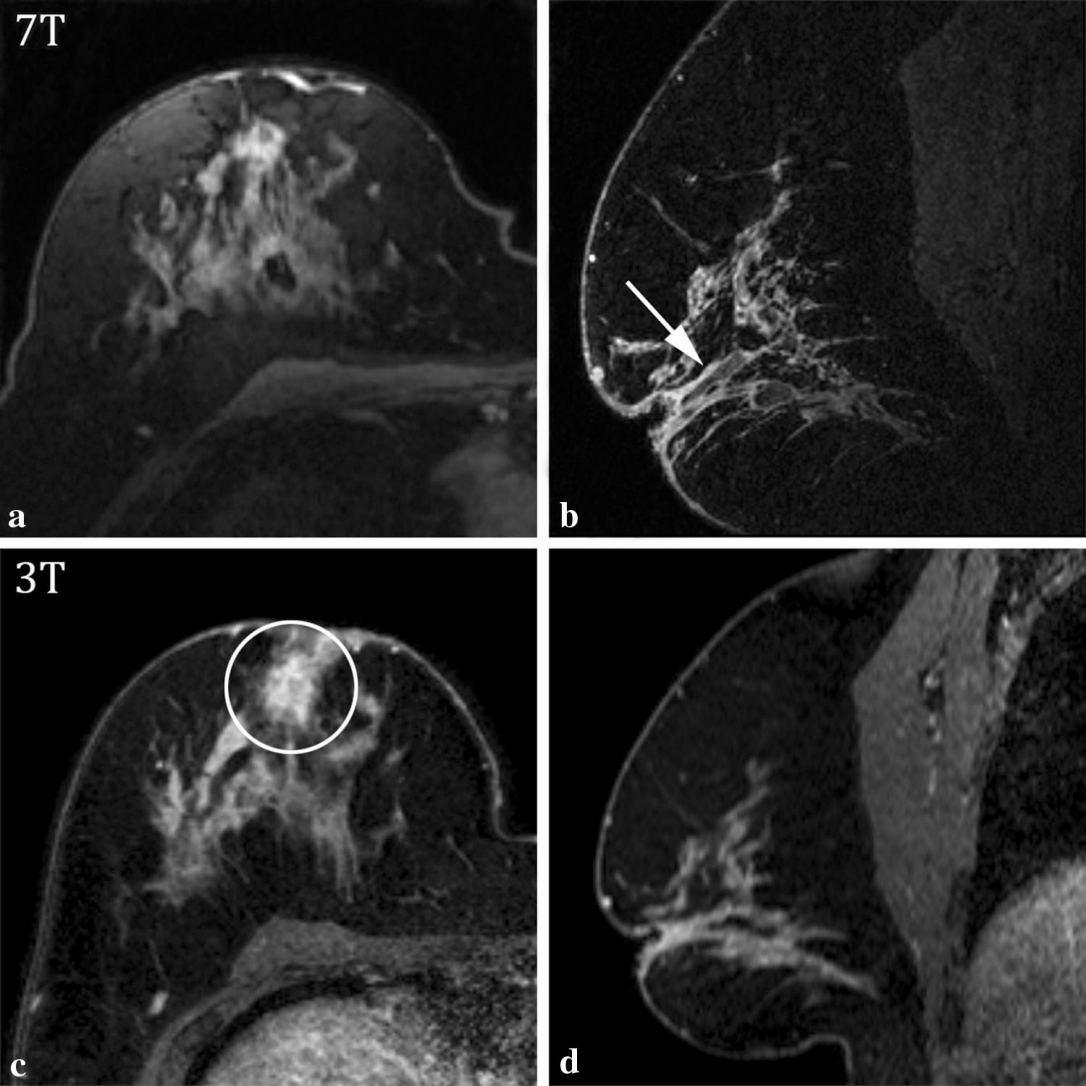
7T (a, b) and 3T (c, d) MRI results of a 47-year-old female patient with a history of inverted nipples. The biopsied index lesion in the right breast showed to be a cyst. Transverse image of 2nd post contrast-injection series (a, c). 7T MRI sagittal slice of high-resolution imaging (b), sagittal slice of 3T dynamic series at approximately the same location (d). At 7T MRI, diffuse non-mass-like enhancement was identified by R2, while R1 identified periductal enhancement (*arrow*). At 3T MRI, a focal non-mass-like enhancement was identified by R1 (*circle*), and multiple regions of non-mass-like enhancement were seen by R2. The observers rate the images BI-RADS 3 for 3T MRI, and BI-RADS 3 (R1) and 2 (R2) for 7T MRI
